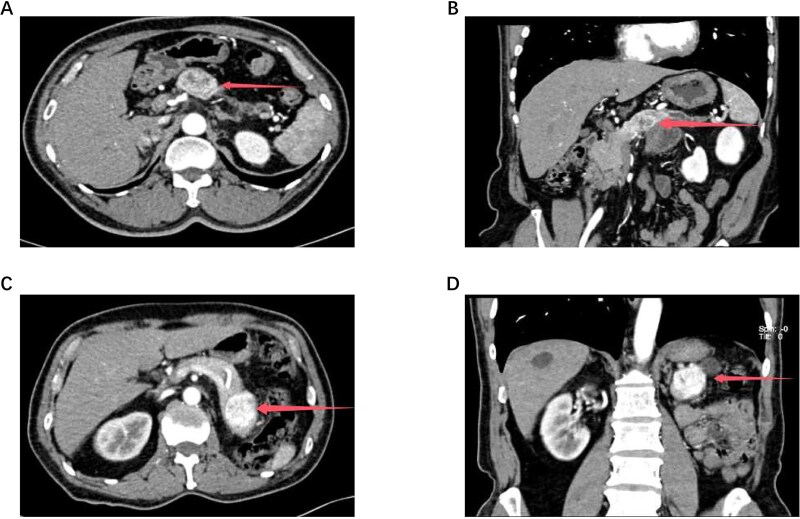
The size and location of the tumors in Cases 1 and 2 are demonstrated by abdominal contrast-enhanced computed tomography (CT) and three-dimensional (3D) reconstruction. Figure 1A shows the tumor in Case 1, and Fig. 1C shows the tumor in Case 2. The corresponding 3D reconstruction results for Cases 1 and 2 are presented in Fig. 1B and Fig. 1D, respectively.
